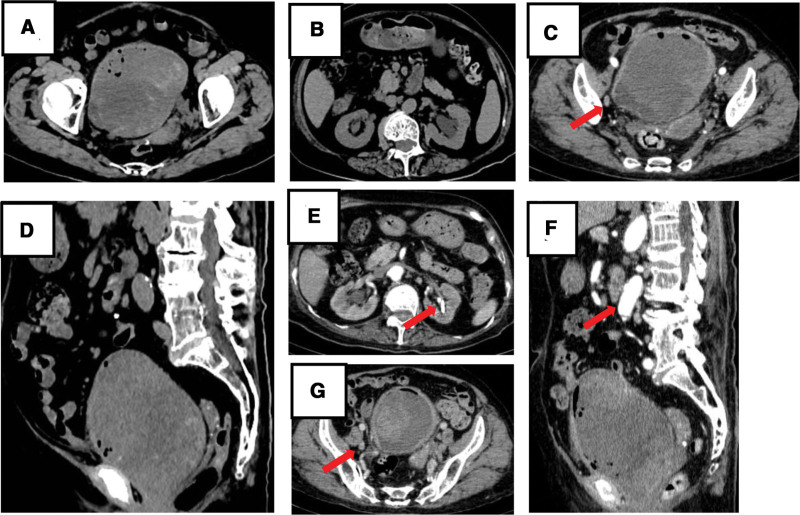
(A, B, and D) show computed tomography (CT) scans (November 17, 2021). (C, E, F, and G) show single-photon emission computed tomography (SPECT) scans (November 25, 2021). (A, D) CT images showing the tumor-filling bladder (Horizontal and sagittal planes). (B) Computed tomography (CT) showing hydronephrosis in the right and left kidneys. (C, G) SPECT showing tumor recurrence and metastasis to the lymph nodes. (Red arrow indicates the right pelvic lymph node.) (E) SPECT image showing bilateral hydronephrosis. (Red arrow indicates the nephrostomy tube previously placed under B-ultrasound guidance) (F) Sagittal view of SPECT. (Red arrows indicate enhancing vessels to confirm that this is a contrast-enhanced scan.)
